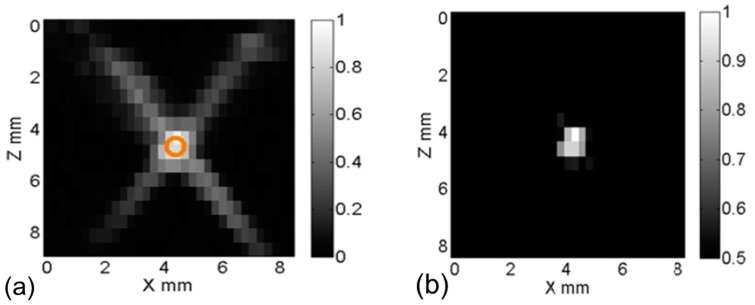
Ultrasound switchable fluorescence images obtained using dual-HIFU for a micro-silicone tube filled with ADP(OH)_2_ based contrast agent; (a) with no threshold applied; and (b) with 50% and above pass through applied.
